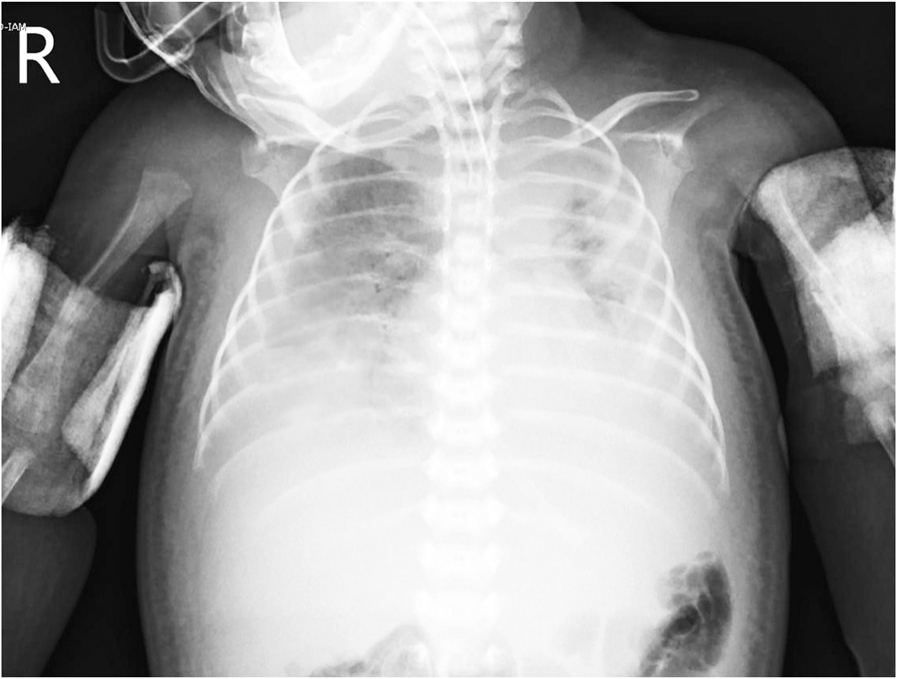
Chest radiograph demonstrated bilateral pleural effusion at 2 weeks of age. Closed fractures of both humeri were noted.
